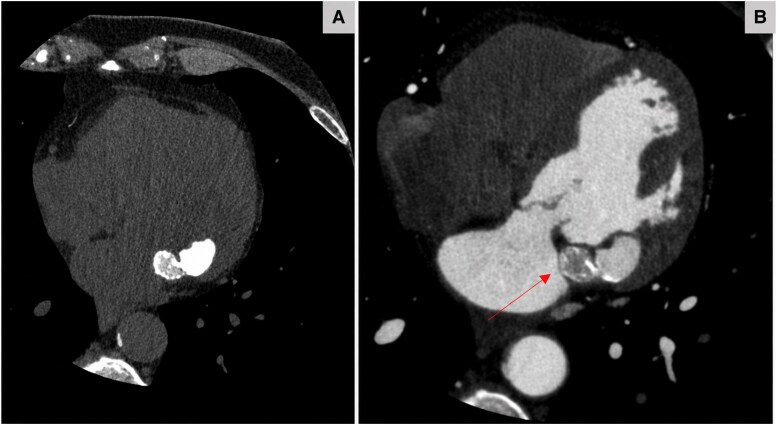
(*A*) Non-contrast CT image showing the marked calcific nature of the posterior mitral annular mass. (*B*) Contrast-enhanced CT showing a well-defined lesion with asymmetric margins and heterogeneous attenuation, including a thin line of calcification (red arrow).
